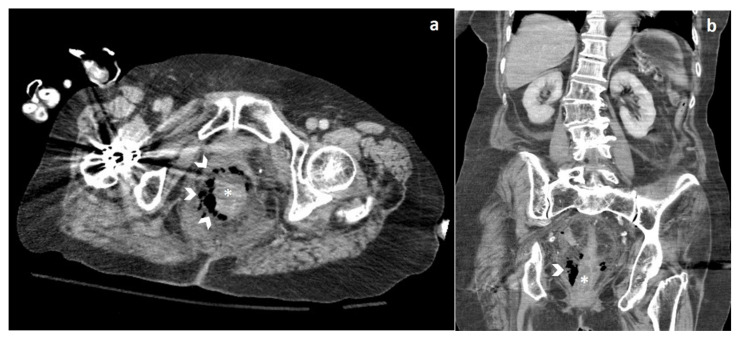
Axial (a) and coronal (b) computed tomography (CT) images show wall thickening (asterisk) of the lower-third of the rectum, surrounded by extraluminal free air (arrowheads).
